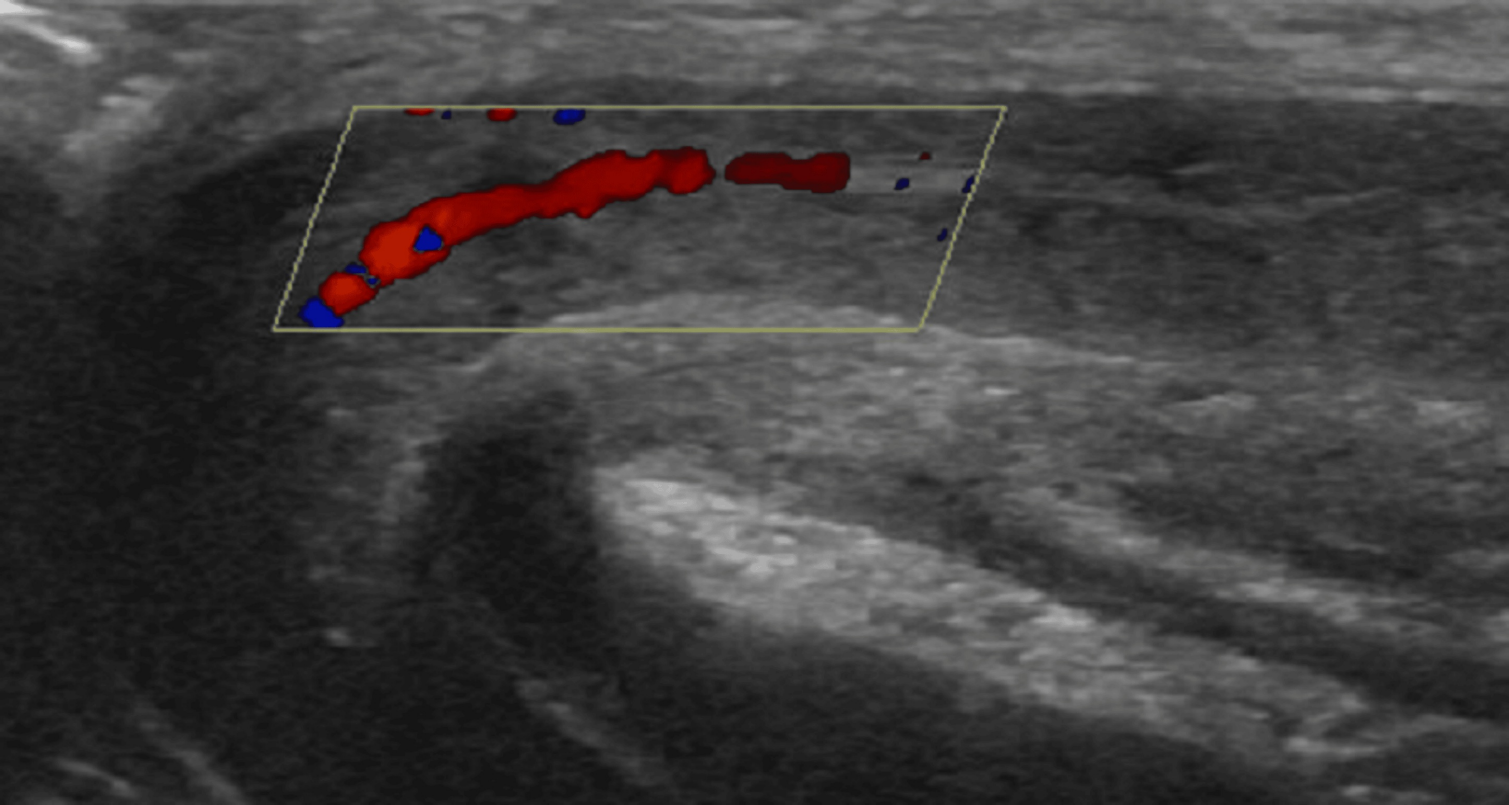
Follow-up duplex ultrasound image at two weeks The observed turbulent, high-velocity flow in the right corpora cavernosa has resolved. Red typically represents arterial flow, and blue indicates venous flow. By six weeks post injury, normal cavernosal artery flow and velocity were observed at the mid to distal shaft, with a small focal heterogeneous area consistent with post-traumatic sequelae
